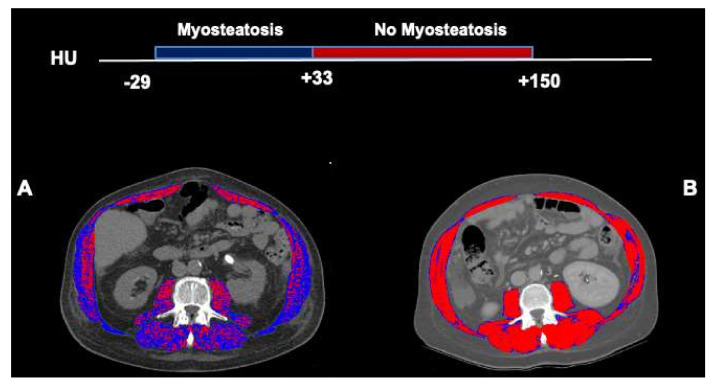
Abdominal computed tomography images taken at the 3rd. lumbar vertebra to quantify muscle radiodensity in patients with cirrhosis. Comparison of two male patients with cirrhosis and similar skeletal muscle index (58 cm^2^/m^2^). Skeletal muscle areas with high radiodensity (33 to 150) are shown in red, and low radiodensity muscle (−29 to 32) is shown in dark blue. In a patient with low mean muscle radiodensity (24 HU) or myosteatosis (A), the majority of the muscle areas are composed of the low attenuation muscle whereas, in a patient with normal muscle radiodensity (51 HU, no-myosteatosis), areas with the normal attenuation range are predominant (B).
